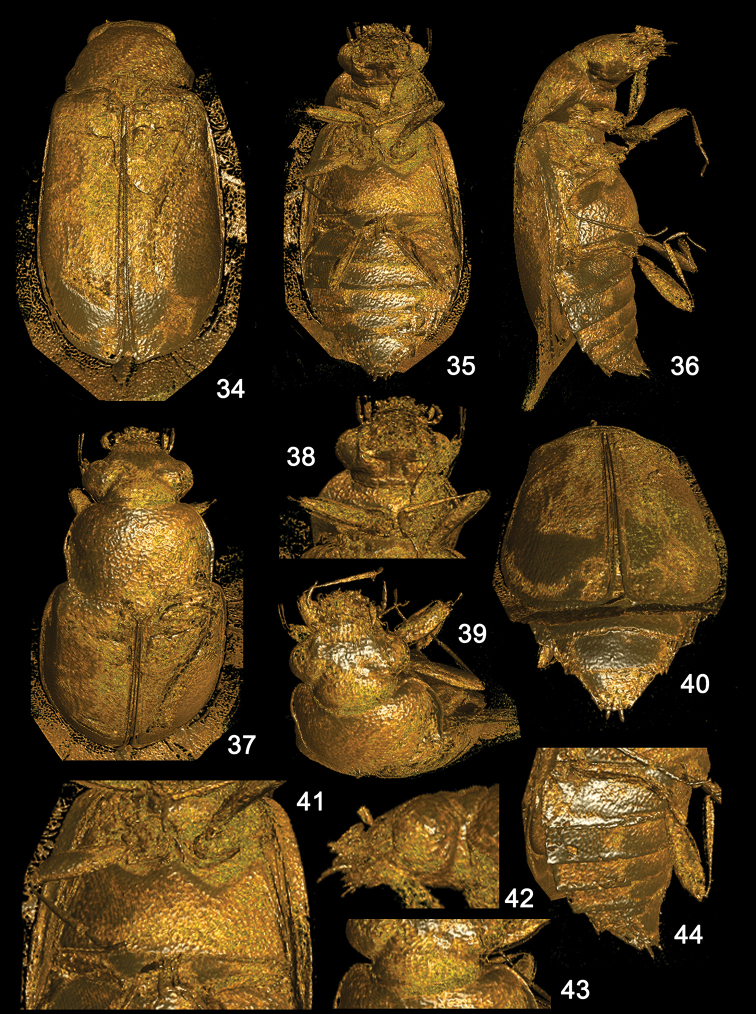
Eusphalerumkanti* sp. nov., paratype, reconstructions from x-ray micro-computed tomography (μ-CT) 34 habitus, dorsal view 35 habitus, ventral view 36 habitus, lateral view 37 forebody, dorsal view 38 head and prothorax, ventral view 39 head and pronotum, anterodorsal view 40 elytra and abdomen, posterodorsal view 41 pterothoracic sclerites, ventral view 42 head, lateral view 43 neck and anterior portion of pronotum, dorsal view 44 abdomen, lateroventral view. Copyright 2015 Shimadzu Corporation.
